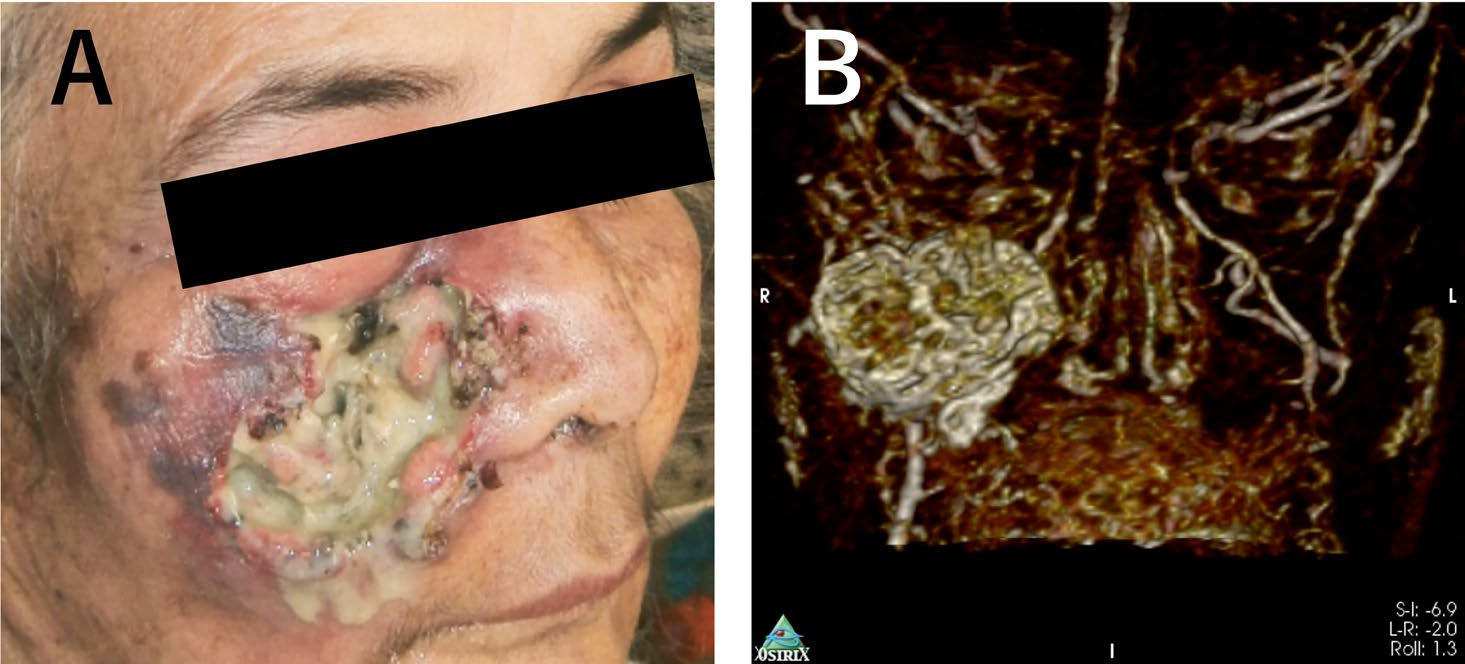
The Picture of wound area and MRI Image. (A) The infection had spread across the upper half of the patient's face during her first visit to the hospital. (B) Image of MRI analysis of the injured site indicated specific findings of wound myiasis.
